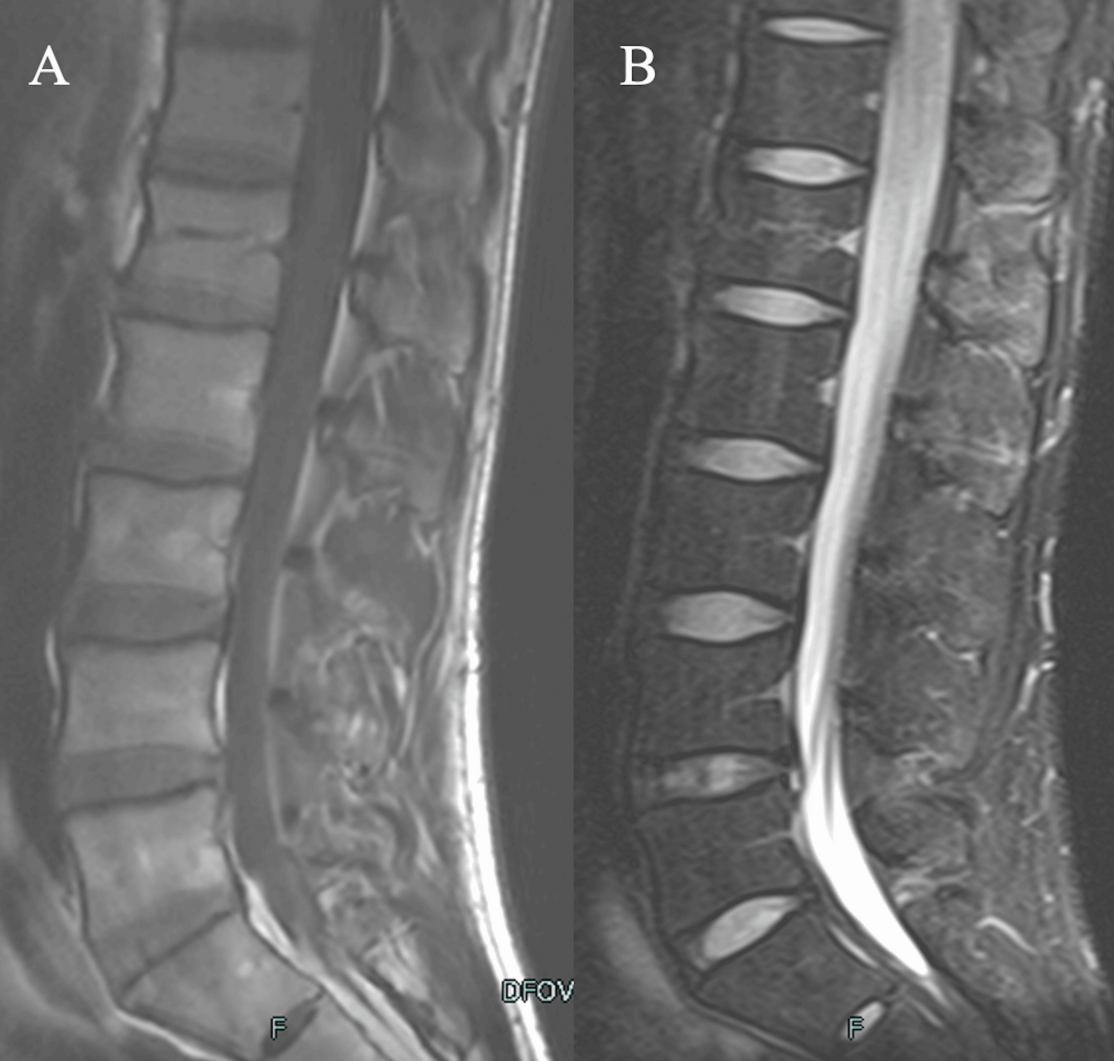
Findings at nine weeks after injury (A) T1-weighted (B) STIR MRI at nine weeks showing resolution of the posterior ligament injury. MRI: magnetic resonance imaging, STIR: short tau inversion recovery
